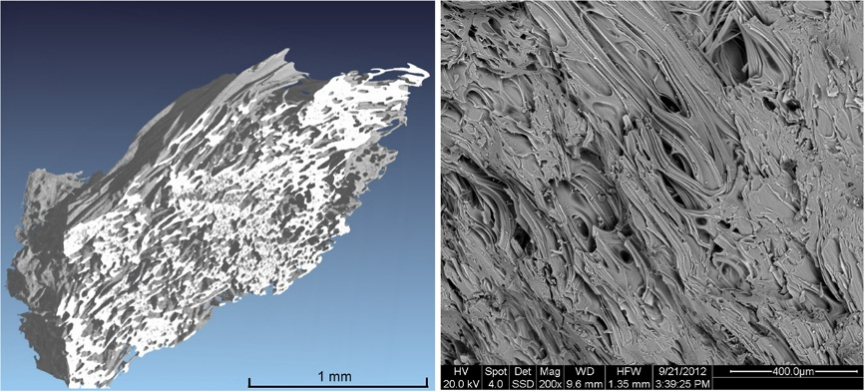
Microstructure of raw perlite visualized by CT (left) and SEM (right).
